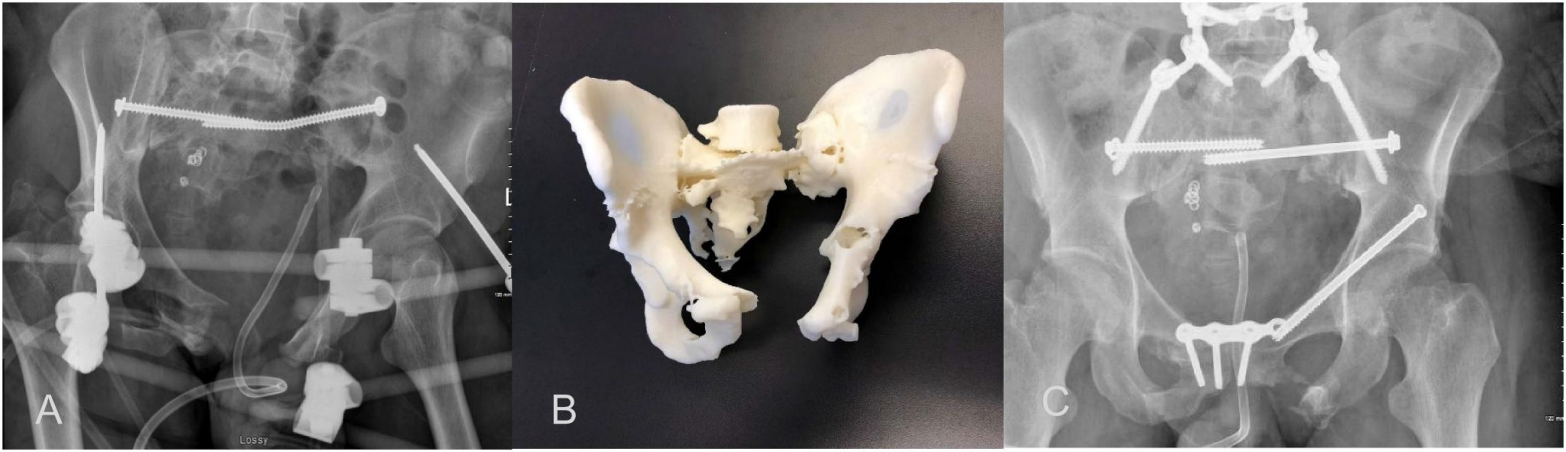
Patient presented with MVA with complex pelvic fracture (A). 3D printing for the pelvis was requested by the trauma team for surgical planning of hardware (B). A successful reconstruction surgery was performed—post operative radiograph (C).
